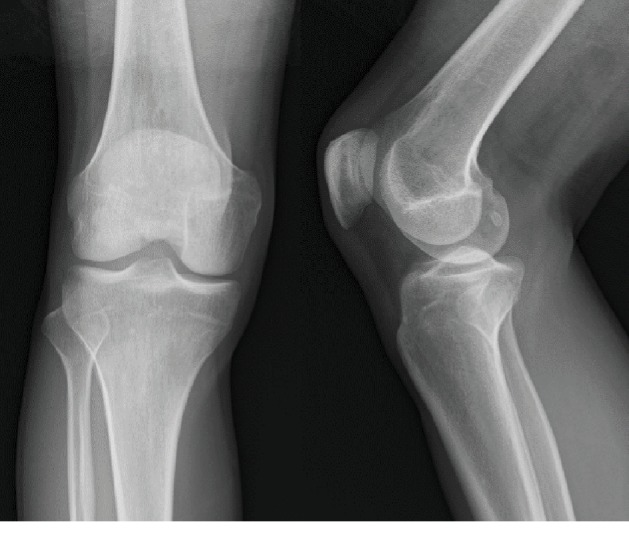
Preoperative radiographs of the knee. Radiographs showed no abnormalities.
